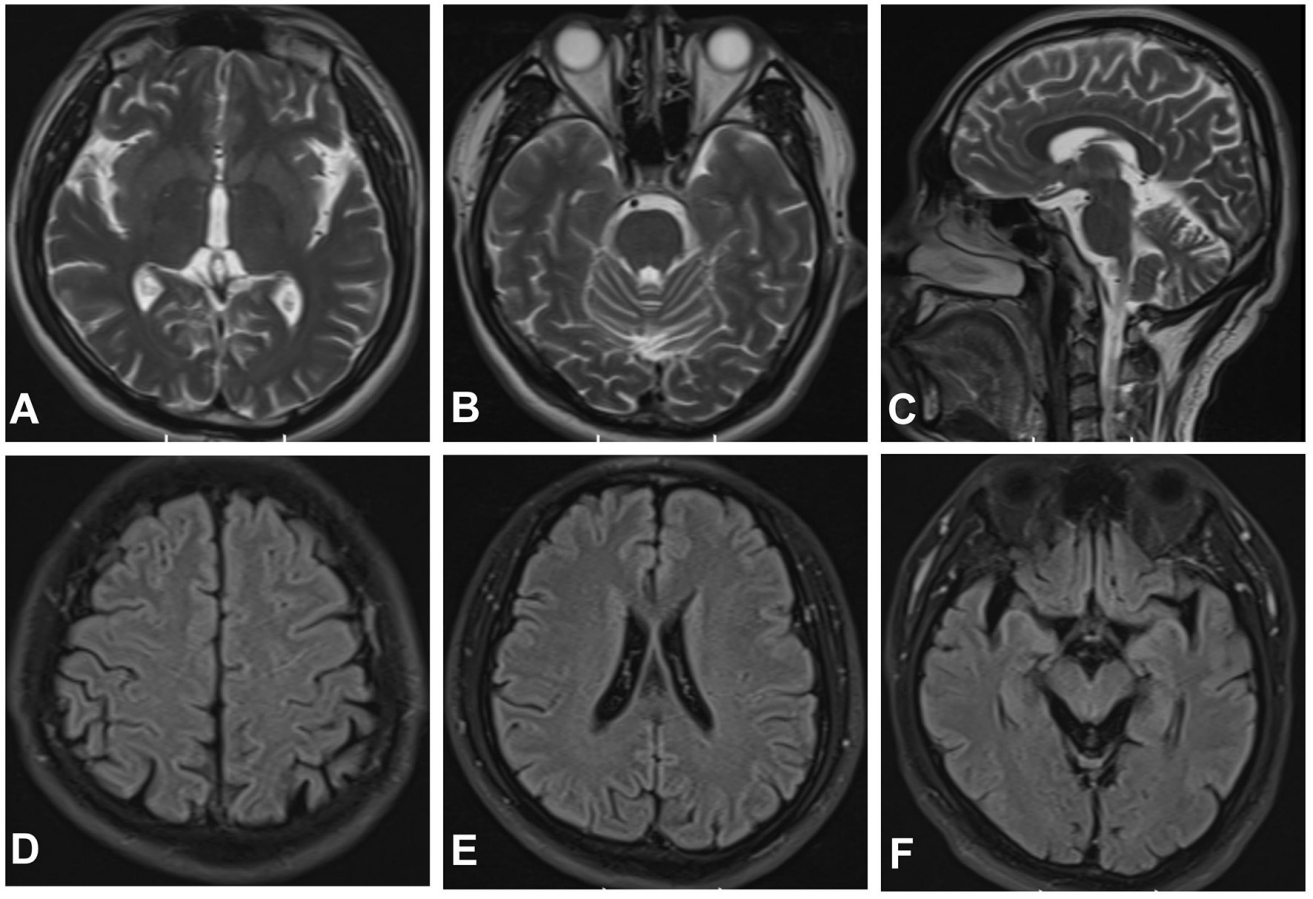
Magnetic resonance imaging (MRI) of the brain. Axial T2-weighted (A, B) and sagittal T2-weighted scan (C) revealed enlarged sulci in the cerebrum. Fluid-attenuated inversion recovery (FLAIR) sequences (D–F) revealed global brain atrophy, ventricular enlargement.
